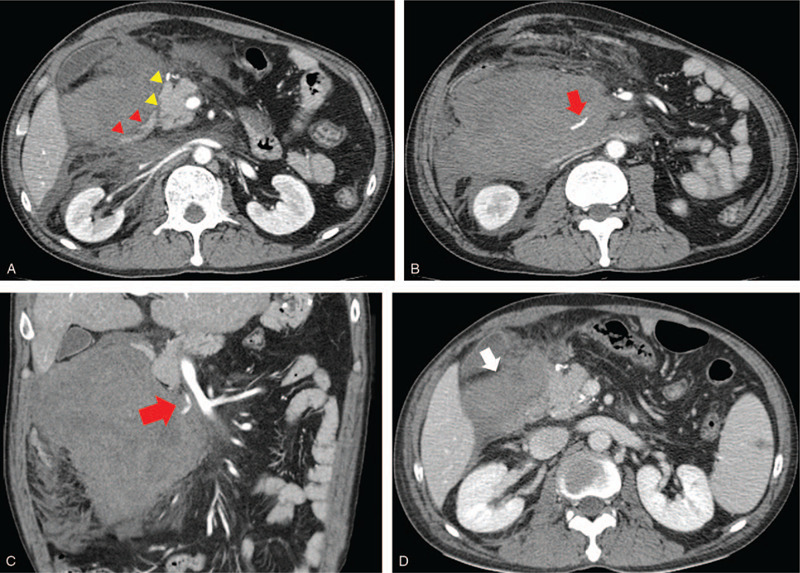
Case 2: a 45-year-old man was admitted to the trauma center after being hit by a car. A, An axial multidetector computed tomography (MDCT) scan revealed a large amount of retroperitoneal hematoma at the right lateral aspect of the pancreas head and second portion of the duodenum. Although they were compressed by a huge hematoma, the margin of the pancreas (yellow arrow head) and the duodenum (red arrow head) are well-preserved. B, An axial MDCT image scanned at the lower level showed active bleeding (red arrow) within the retroperitoneal hematoma. C, The coronal MDCT scan showed that the active bleeding (red arrow) was located between the pancreas head and the superior mesenteric vessels. D, Follow-up MDCT scan 7 days after transcatheter arterial embolization (TAE) revealed an old hematoma at the right lateral aspect of the pancreas head and it was smaller than before TAE.
